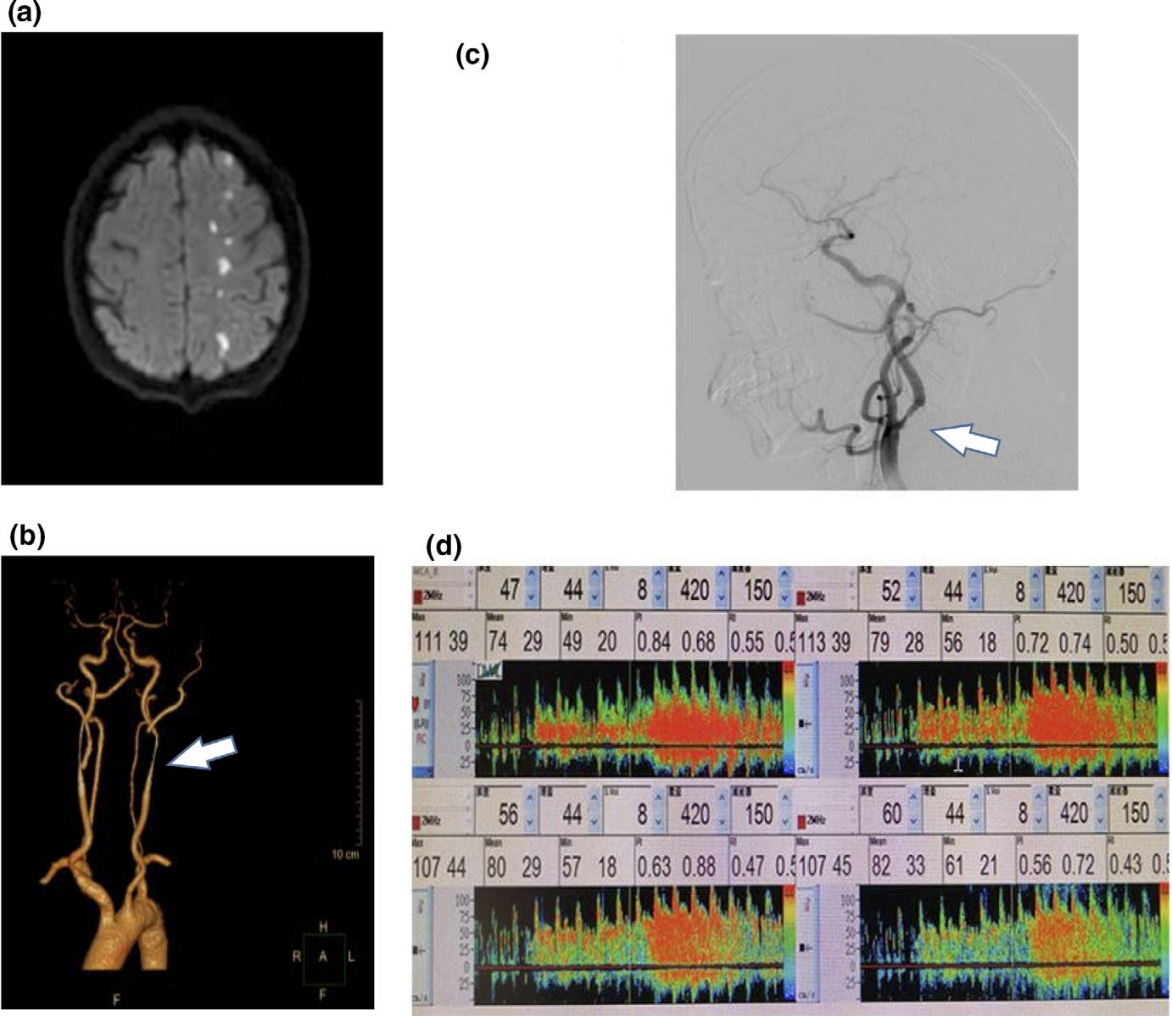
Artery‐to‐Artery embolism stroke mechanism in ischemic stroke patients with ICAS. Images from a 77‐year‐old woman with acute cerebral infarction in the left cerebral hemisphere. When the patient was admitted to the hospital, the consciousness was clear, presented with the right limb muscle strength was level 4, and the left limb muscle strength was level 5. NIHSS on admission was 2, and mRS at discharge was 1. (a) DWI shows small, scattered, cortical embolic infarcts in left hemisphere indicating probable artery‐to‐artery embolism. (b, c) CTA and DSA showed left internal carotid artery sinus ulcer plaque and severe stenosis. The arrows indicate stenosis location. (d) Transcranial doppler ultrasonography can detect symptomatic or asymptomatic embolism during microembolic signal monitoring
